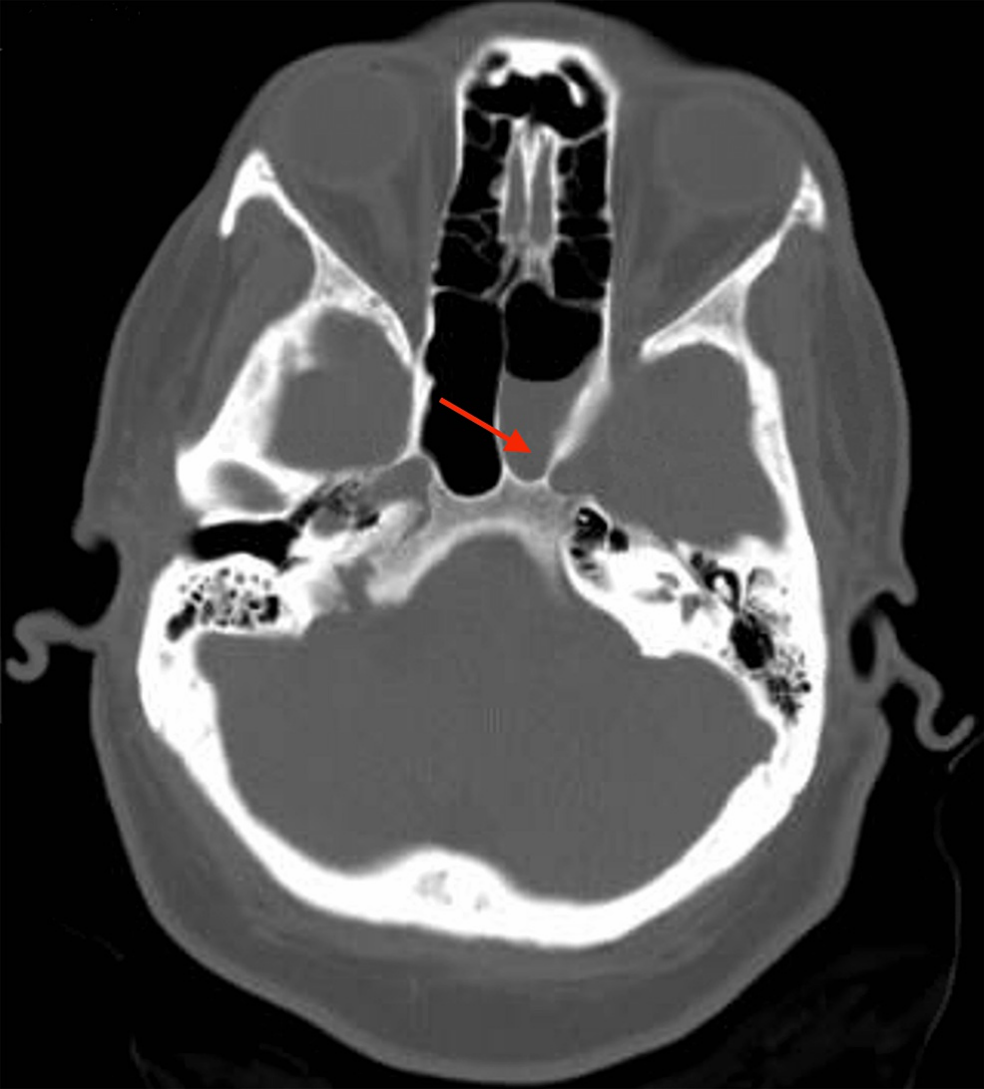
Axial computed tomography (CT) of the head and sinus Axial CT of the head and sinus depicts increased opacification (red arrow) in the left sphenoidal sinus
